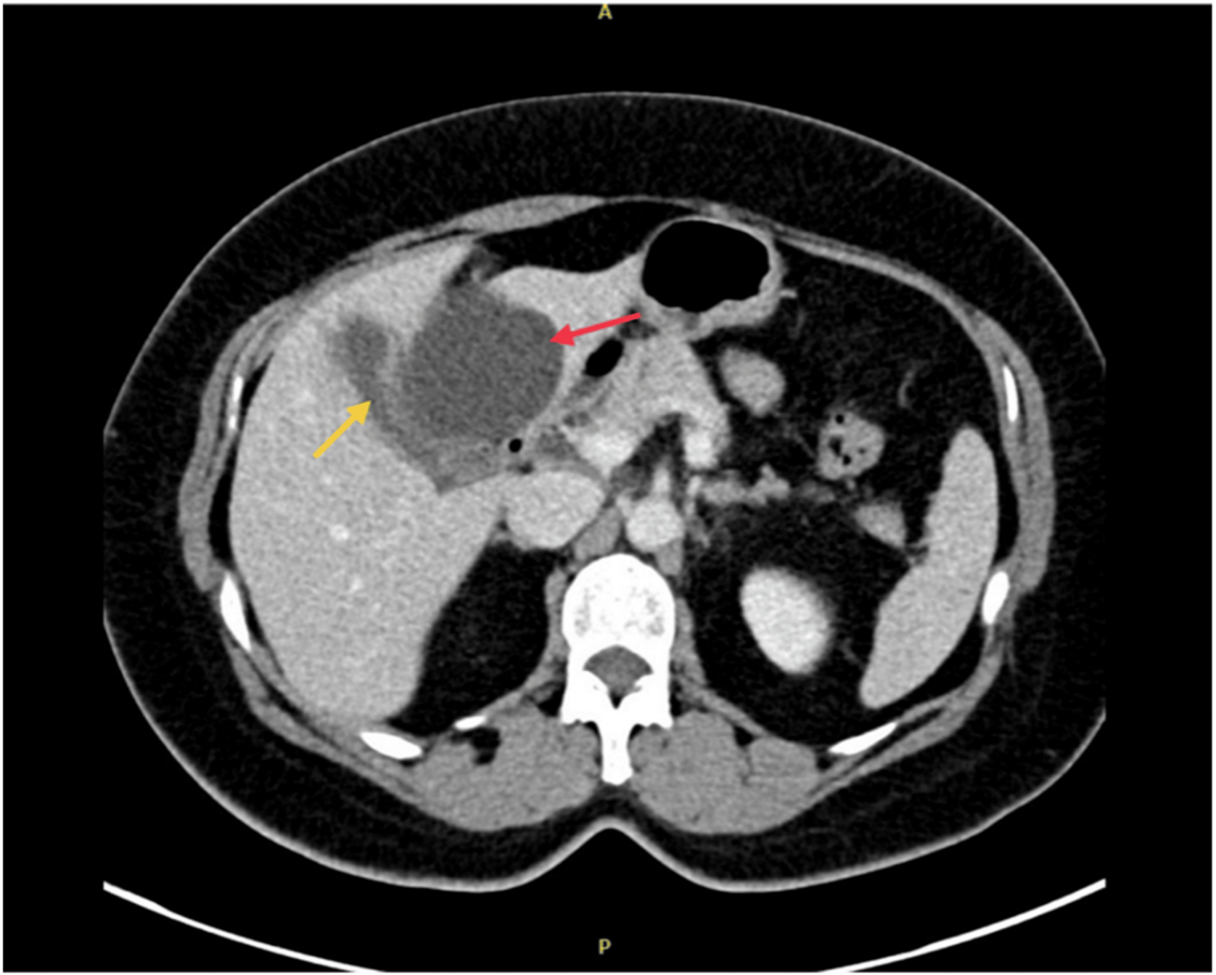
Supplemental CT image cross-section displaying visual obstruction of the gallbladder (yellow arrow) by a dominant left lobe cyst (red arrow)
